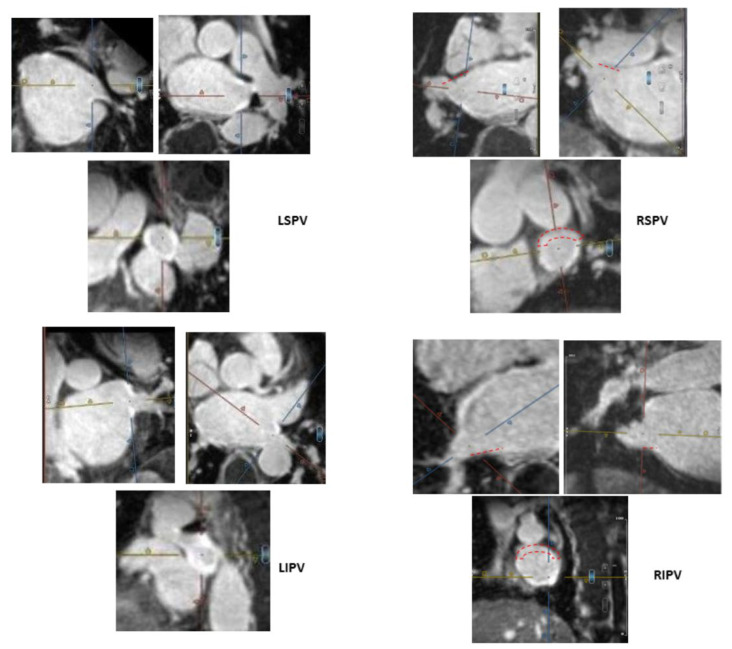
Cross-sectional short-axis views of PVs on post-ablation LGE MRI using the “double oblique” technique. For each PV, two orthogonal plane images are used to create its circular cross-sectional short-axis view. (PV are centered between the MRI orthogonal lines.) Each PV ostium was divided into four segments: anterior, posterior, roof, and floor. Based on the cross-sectional views of PV ostia, ablation-induced PV ostial fibrosis are categorized as complete circumferential fibrosis, sub-complete circumferential fibrosis with single “minor” gap defined as LGE gap ≤ 1/3 PV segment length, partial fibrosis with major gaps defined by as LGE gap > 1/3 segment length, or absent PV fibrosis. In this patient, both left PVs are fully encircled by LGE enhancement (seen as bold white lines), defined as complete PV circumferential fibrosis, while both right PVs have major gaps (
